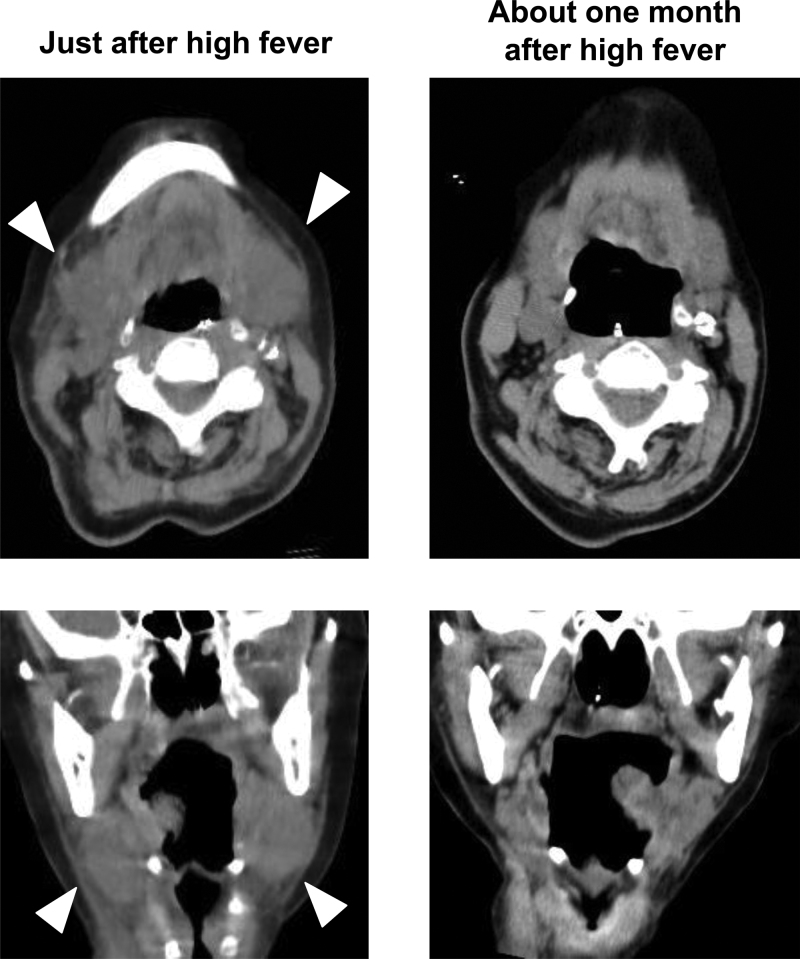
Cervical computed tomography (CT) after high fever in this subject receiving parenteral nutrition with nasogastric tube feeding for 22 days in our hospital. Cervical CT revealed swelling of the bilateral submandibular glands and fluffing of surrounding tissue (white arrowhead) (left panel). Cervical CT about 1 month after high fever showed that enlargement of submandibular gland was drastically improved receiving parenteral nutrition with nasogastric tube feeding for 57 days (right panel).
